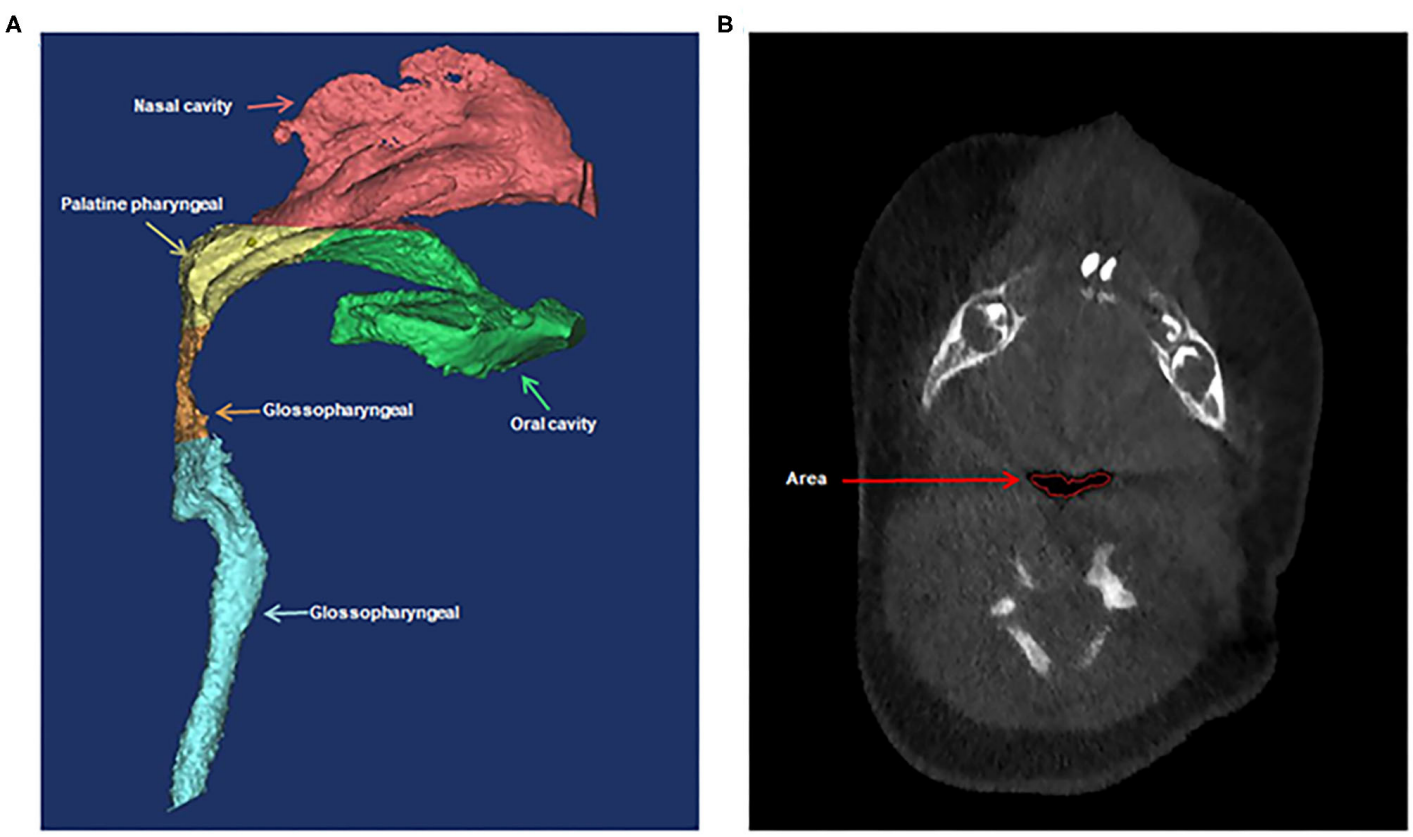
Three-dimensional computed tomographic measurements of the airway volumes (A) and area (B). (A) The palatine pharyngeal airway from the posterior border of the hard palate to the edge of the soft palate and the glossopharyngeal airway from the tip of soft palate to the upper edge of epiglottis. (B) The airway section area at the tip of epiglottis.
